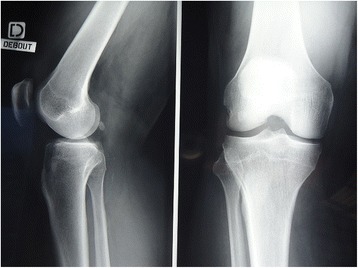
Preoperative radiography. Anterior-posterior and lateral view. No sign of arthritis on the medial compartment
